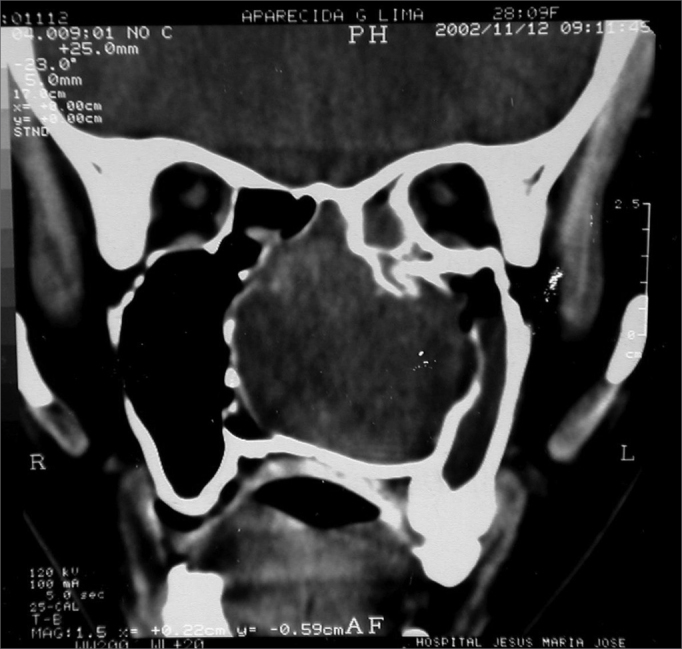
CT scan - coronal view of a neurofibroma (case 5).
